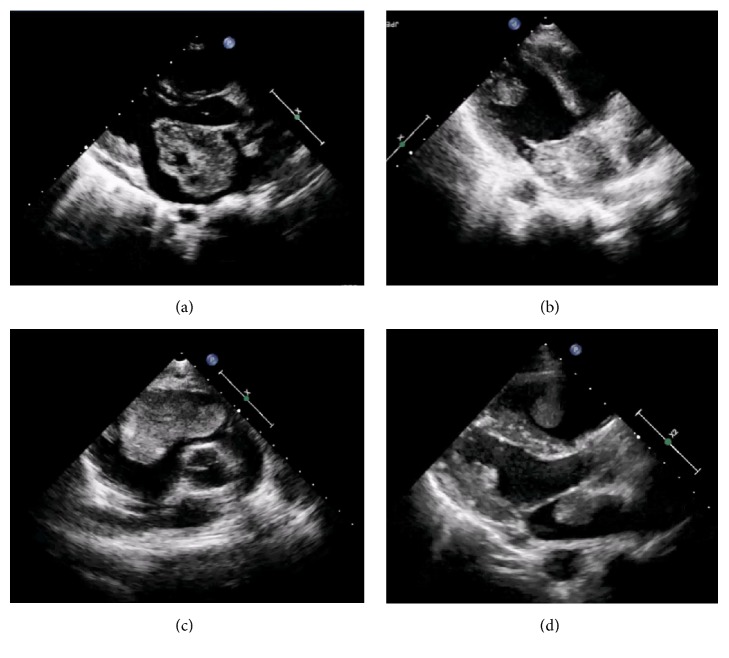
Still images of echocardiogram form the initial diagnosis and subsequent recurrent CM, panel (a) showing a LA mass during initial diagnosis; panel (b) showing LA and LV masses in the first recurrent episode; panel (c) showing an RV mass in the second recurrent episode; panel (d) showing LA, LV, and RV masses in the third recurrent episode.
